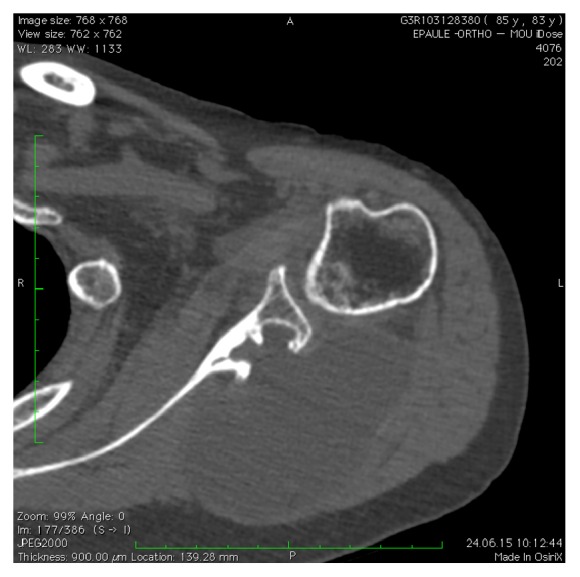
CT scan confirming the invasion of the scapula by the mass.
